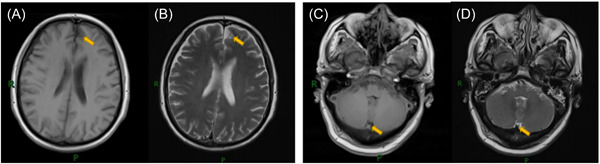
Brain magnetic resonance imaging scan + enhancement: bilateral frontal ischemic lesions (A, B). Bilateral parietal and tentorial meninges thickening and enhancement (C, D). The following possibilities are considered: intracranial hypotension syndrome, meningitis, idiopathic hypertrophic pachymeningitis, and so on. The pituitary gland is plump. [Color figure can be viewed at wileyonlinelibrary.com]
